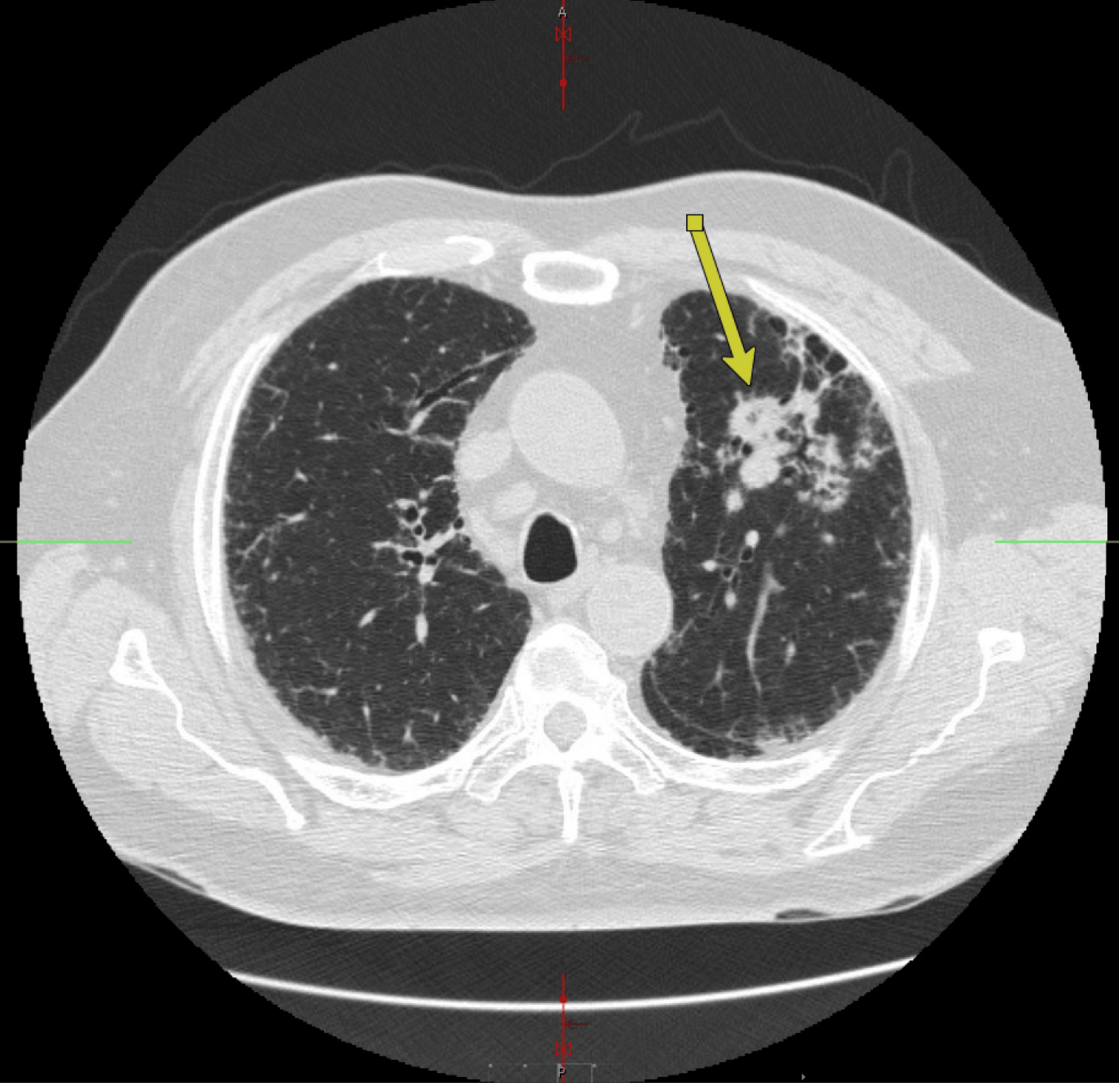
High-resolution CT Chest: conglomerative solid mass-like opacity/clustered nodular opacities in the anterior left upper lobe
